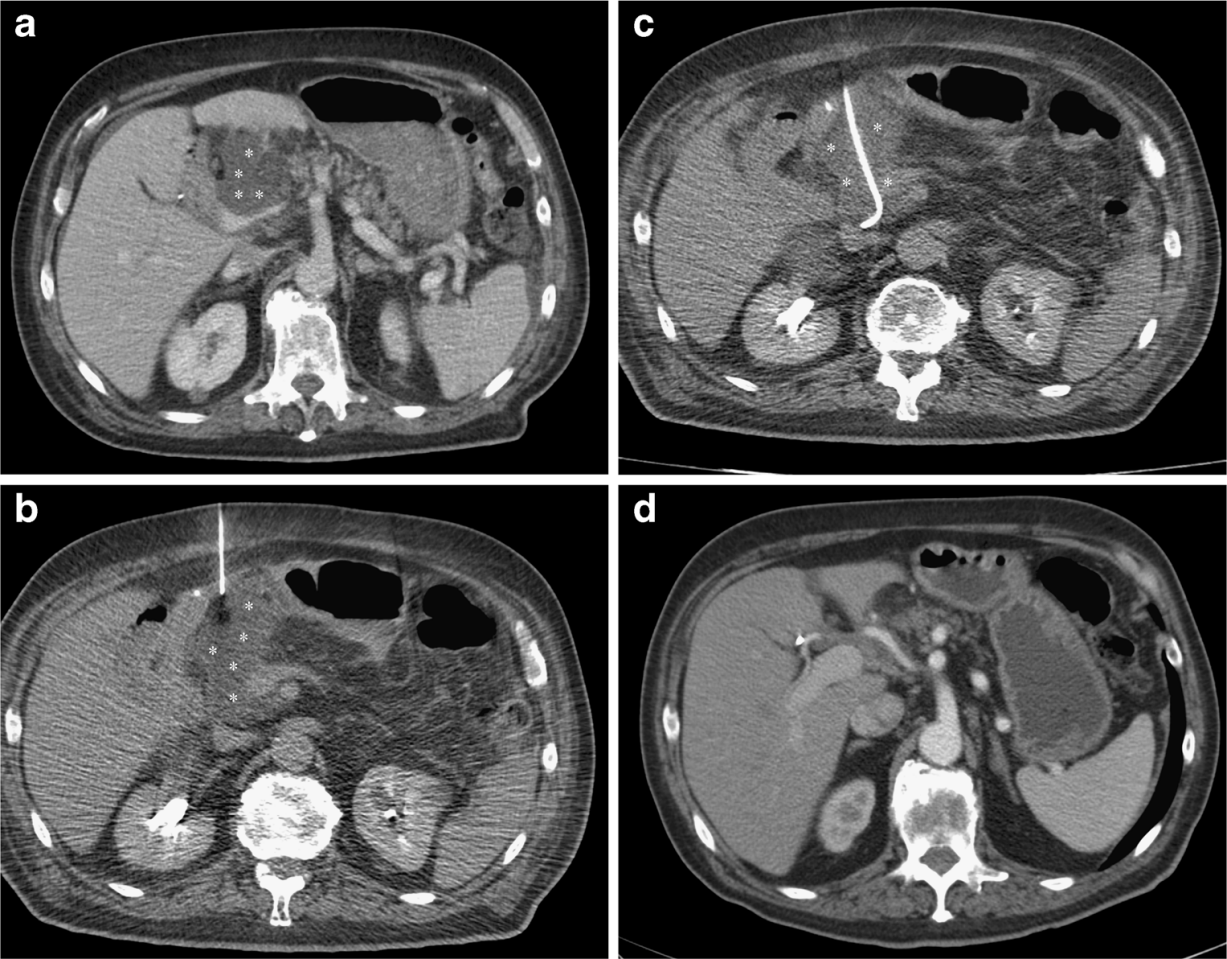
Use of a percutaneous drainage to treat a post-pancreaticoduodenectomy fluid collection. a CT scan shows post-pancreaticoduodenectomy retroperitoneal fluid collection (*asterisks*). b The fluid collection (*asterisks*) is punctured with a small needle under CT guidance. c A percutaneous drainage (*arrow*) is inserted into the collection (*asterisks*) using the Seldinger technique. d At the end of the treatment complete resolution of the collection is obtained
